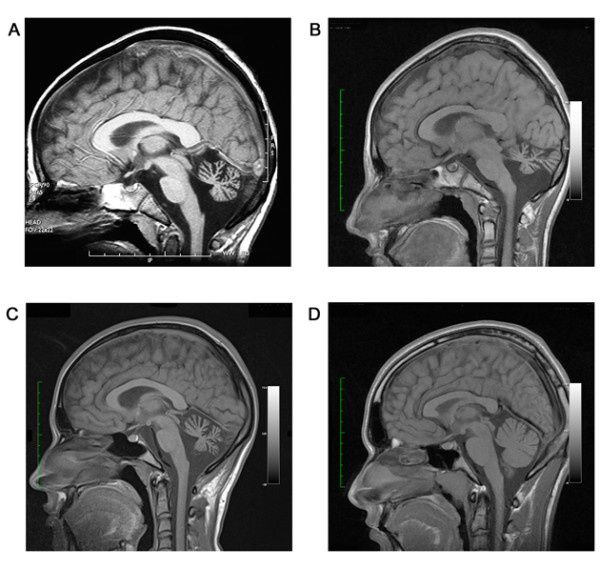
Brain T-1 weighted magnetic resonance imaging showing marked cerebellar atrophy in P1 at the age of 9 years (panel A) and 14 years (panel B), and in P2 at the age of 18 years (panel C). Panel D shows a normal brain MRI in a 16 years-old control.
